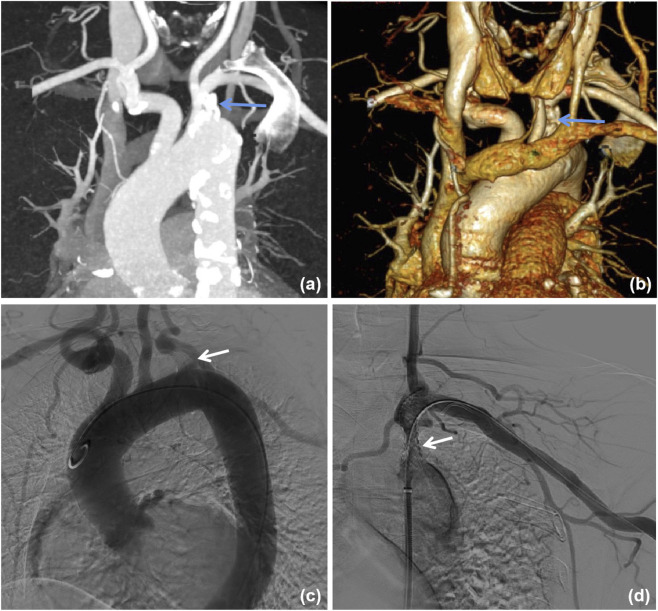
Images of subclavian artery stenosis. (a) Computed tomography angiography shows severe stenosis at the origin (arrow). (b) Stenosis (arrow) in 3D reconstruction. (c) Digital subtraction angiography shows occlusion at the origin of the subclavian artery (arrow). (d) Successful resolution of the stenosis following percutaneous transluminal angioplasty and stenting (arrow).
